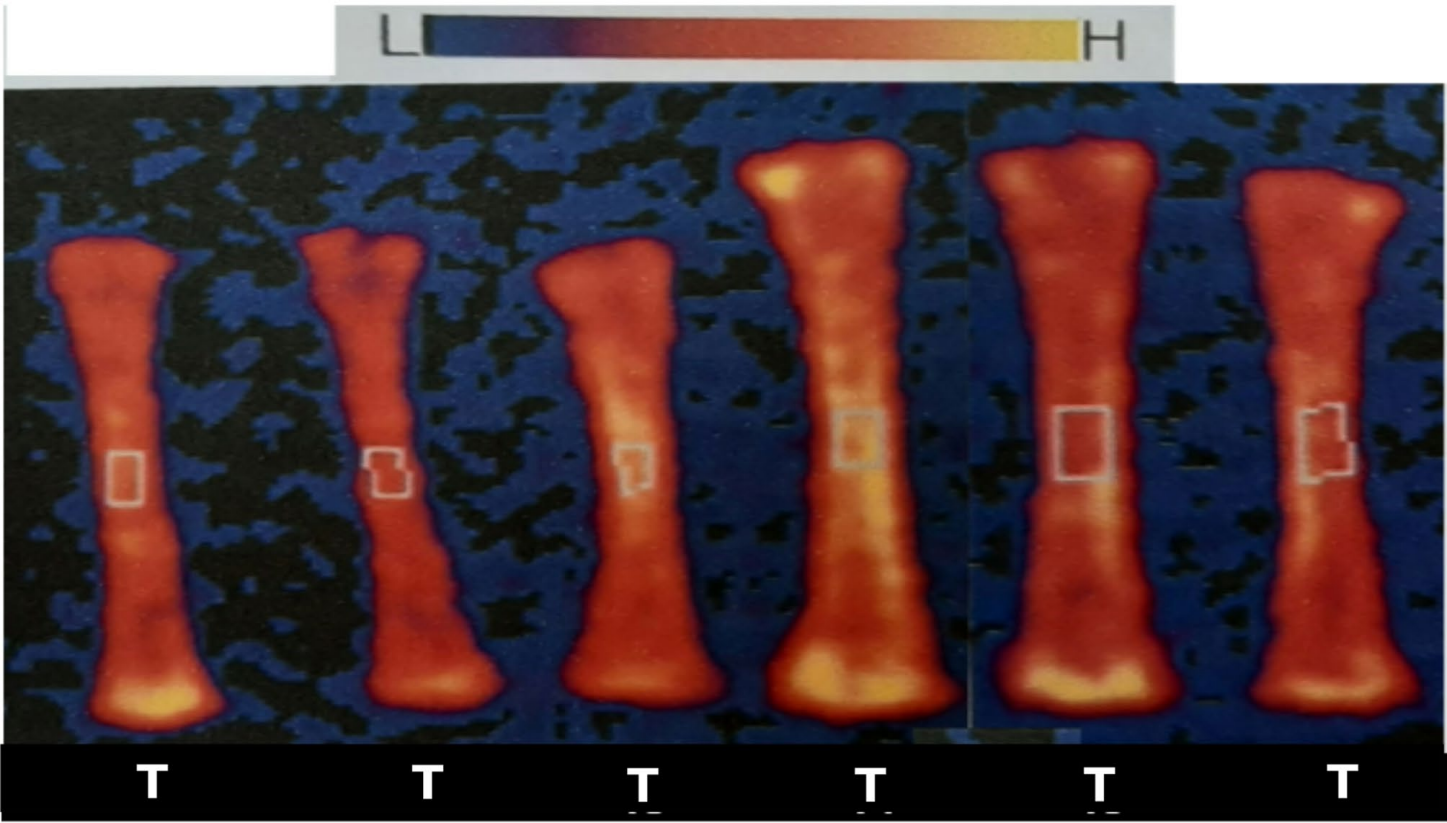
Radiographic image of the effect of different treatments on tibia bone scans using dual energy X-ray absorptiometry at 35 days of age.
